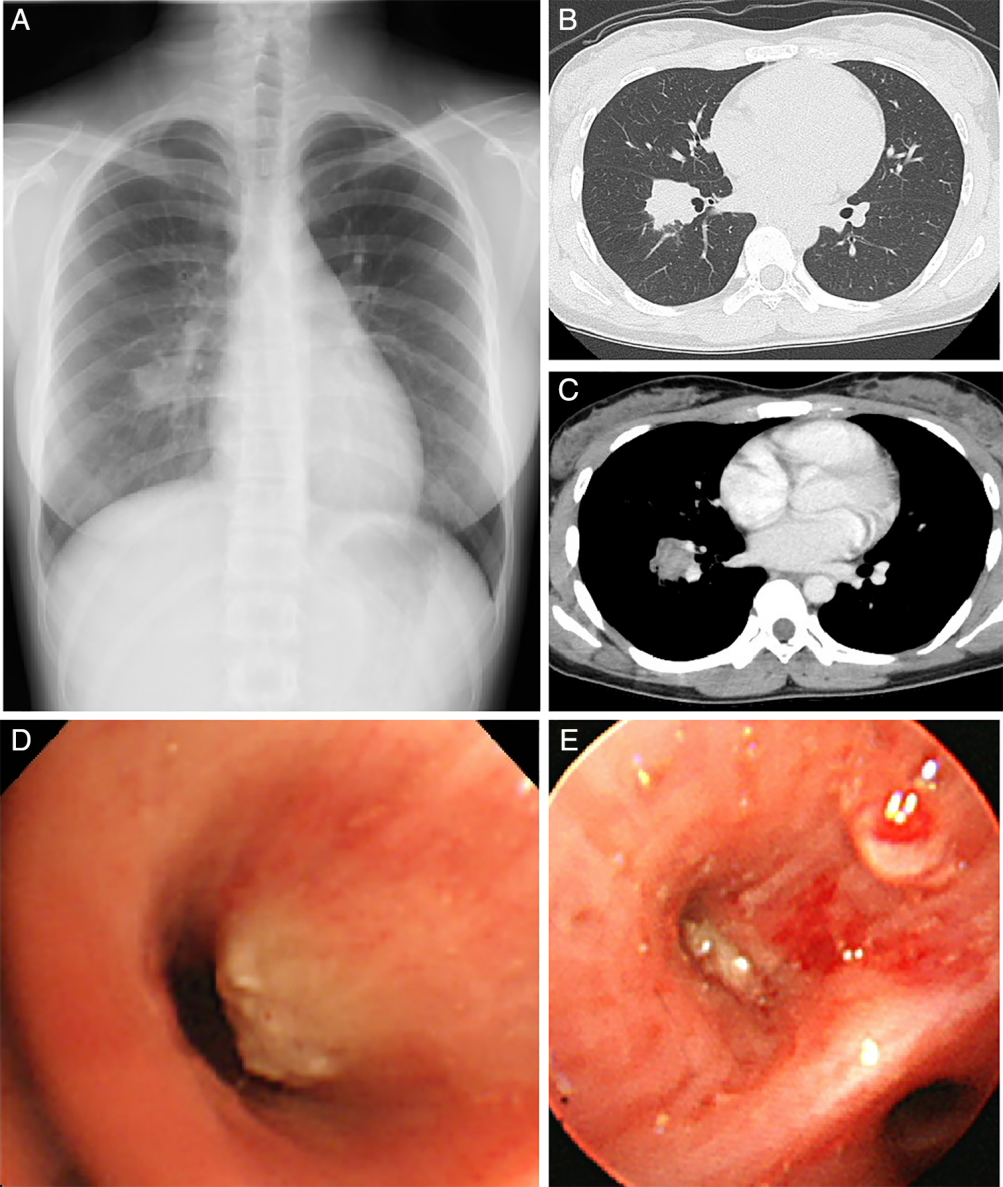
Chest X‐ray on the patient’s first visit to our hospital demonstrated a nodule as large as 3 cm in diameter in the right middle lung fields (A), which was confirmed by contrast‐enhanced thoracic computed tomography as an inhomogeneously enhanced solitary nodule measuring 3 cm in size at the proximal portion of B8/B9 (B, C). Repeated bronchoscopy was performed at 10 hospital days (D) and four weeks (E) after the first visit to our hospital. The tumour compressed the tracheal lumen (D) and then entirely occluded the B8.
